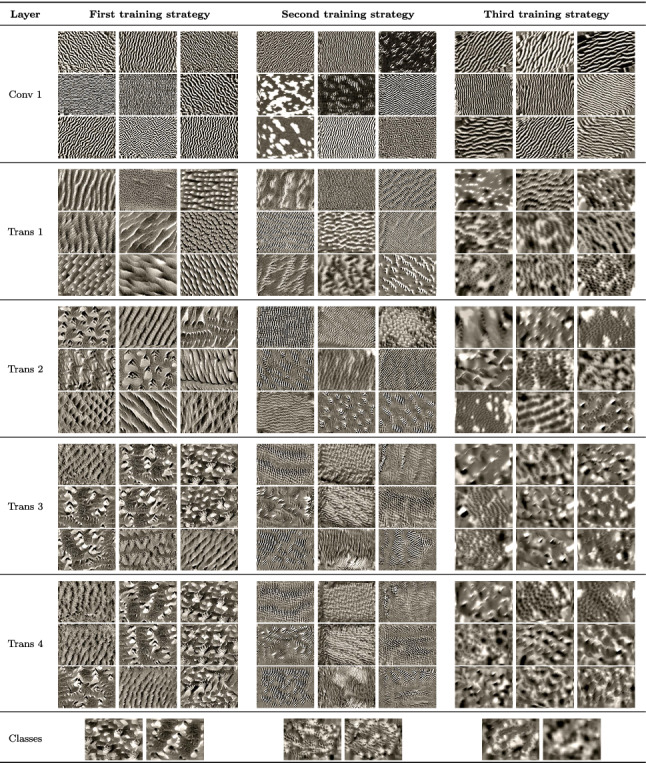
Feature visualization for single channels in CovidDenseNet trained on the SARS-CoV-2 CT-scan dataset with different training strategies. Best viewed in color with zoom
